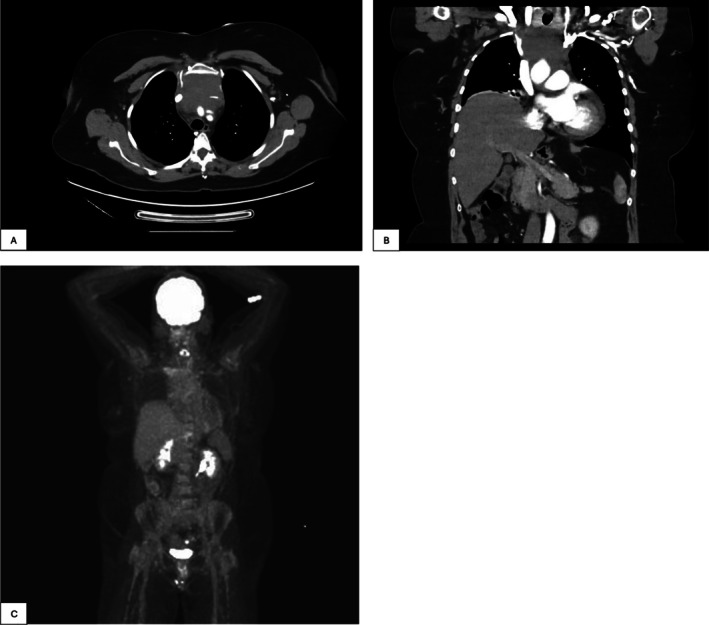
Initial CT and FDG PET imaging. (A) Initial CT: axial view. The tumor measures up to 6.0 cm in the anterior–posterior dimension. (B) Initial CT: coronal view. The tumour measures up to 7.5 cm in the transverse dimension and 6.0 cm in the superior–inferior dimension. (C) Initial PET: Maximum intensity projection.
